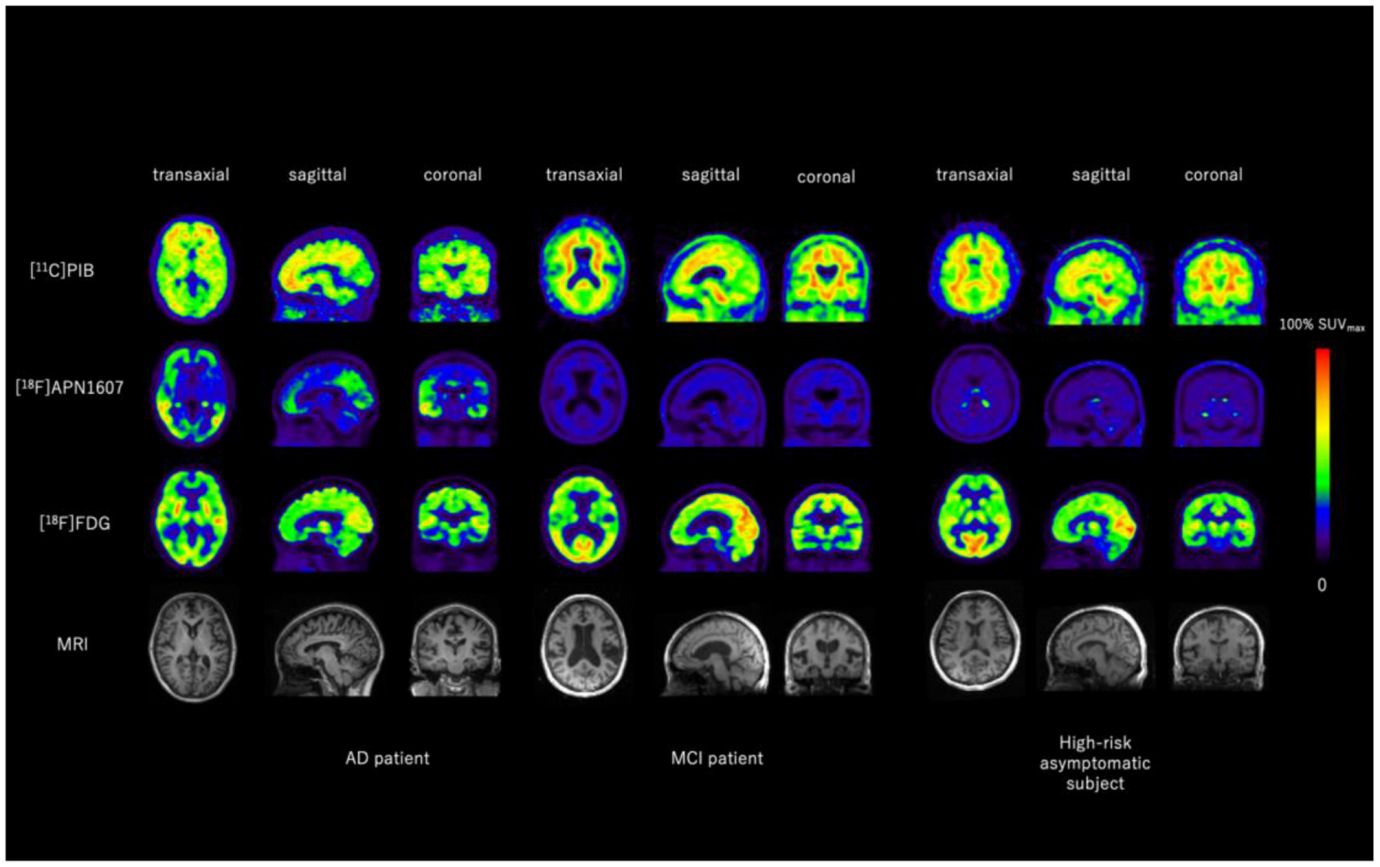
Representative images of [^11^C]PIB, [^18^F]APN1607, and [^18^F]FDG PET and MRI of an AD patient, an MCI patient and a high-risk asymptomatic subject. Aβ was positive for the AD patient and equivocal for the MCI patient and the high-risk asymptomatic subject. Typical Braak Stage V–VI tau deposition was seen in the AD brain, whereas no tau deposition was seen in the brain of the MCI patient or the high-risk asymptomatic subject. Typical AD-like glucose-hypometabolism was seen in AD brain including posterior cingulate cortex, parietal, temporal, and pre-frontal cortices, whereas most of these regions are relatively spared in the brain of MCI patient and high-risk asymptomatic subject. Images provided by PET Center, Huashan Hospital, Fudan University.
